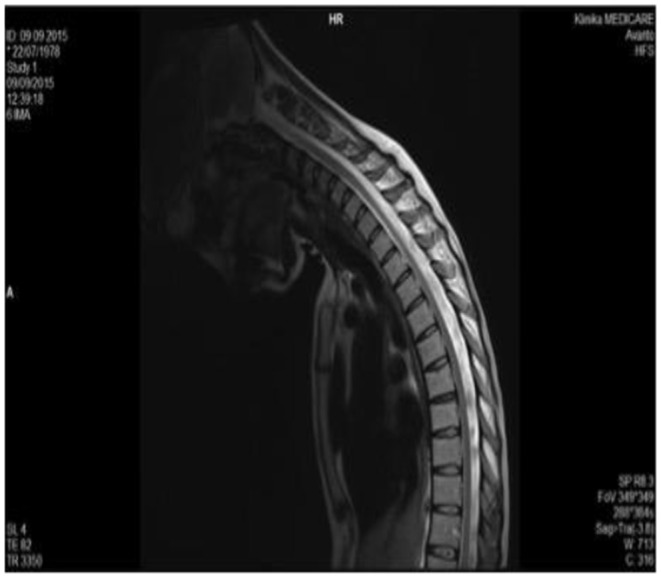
Cervical MRI of the neck in antero flexion, which does not show the hallmark of HD, i.e., the forward movement of the posterior wall of the inferior cervical dural sac (2015).
